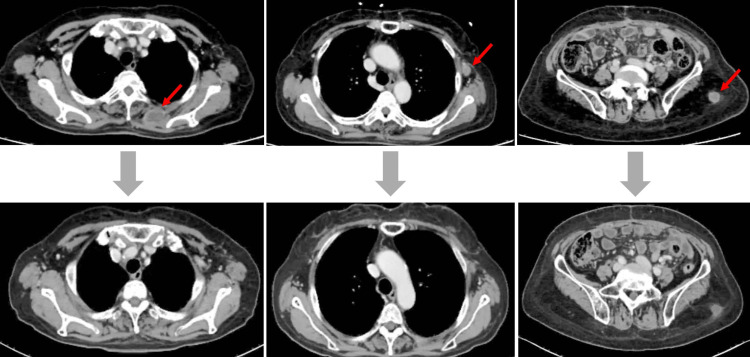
CT images showing response to chemotherapy The upper row displays pre-treatment images, while the lower row presents post-treatment images following three cycles of paclitaxel and carboplatin. A nearly complete resolution of all metastatic lesions is observed. Red arrows indicate subcutaneous metastases CT: computed tomography
